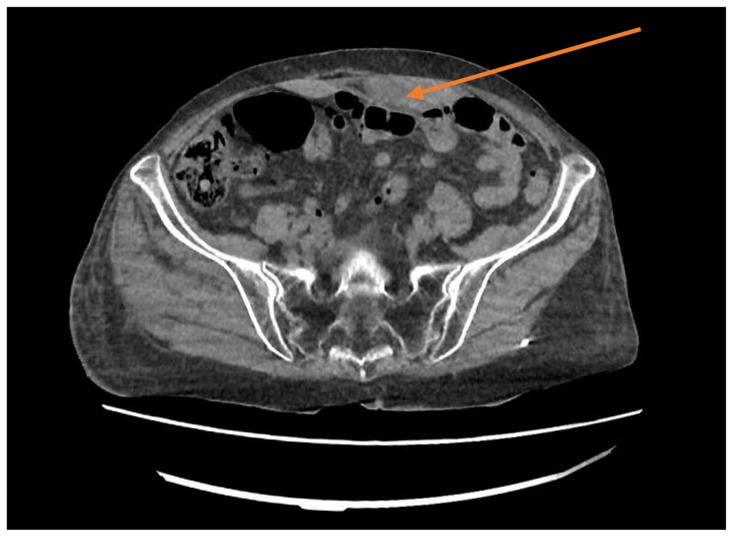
CT scan showing an abdominal mass in the left rectus sheath (orange arrow).
